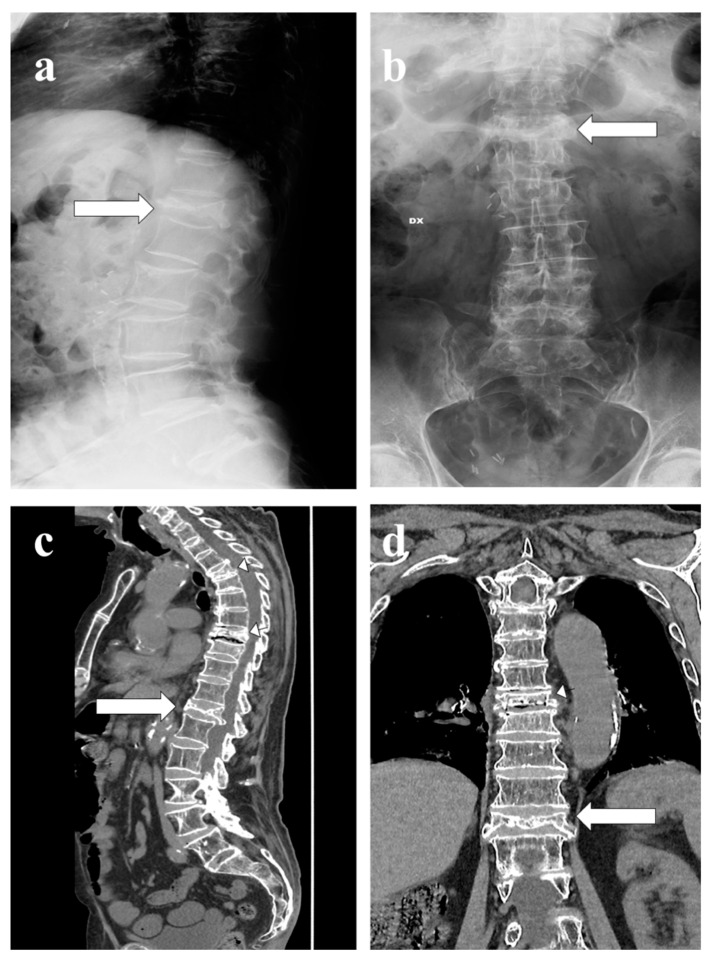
Vertebra plana in metastatic carcinoma. An 87-year-old female was treated 2 years before with resection of the sigma for colorectal carcinoma. (a) lateral and (b) anteroposterior radiographs show clear vertebra plana at the T12-level (white arrow). CT scan in (c) sagittal and (d) coronal view show multiple pathologic fractures (arrowheads) in the thoracic spine.
